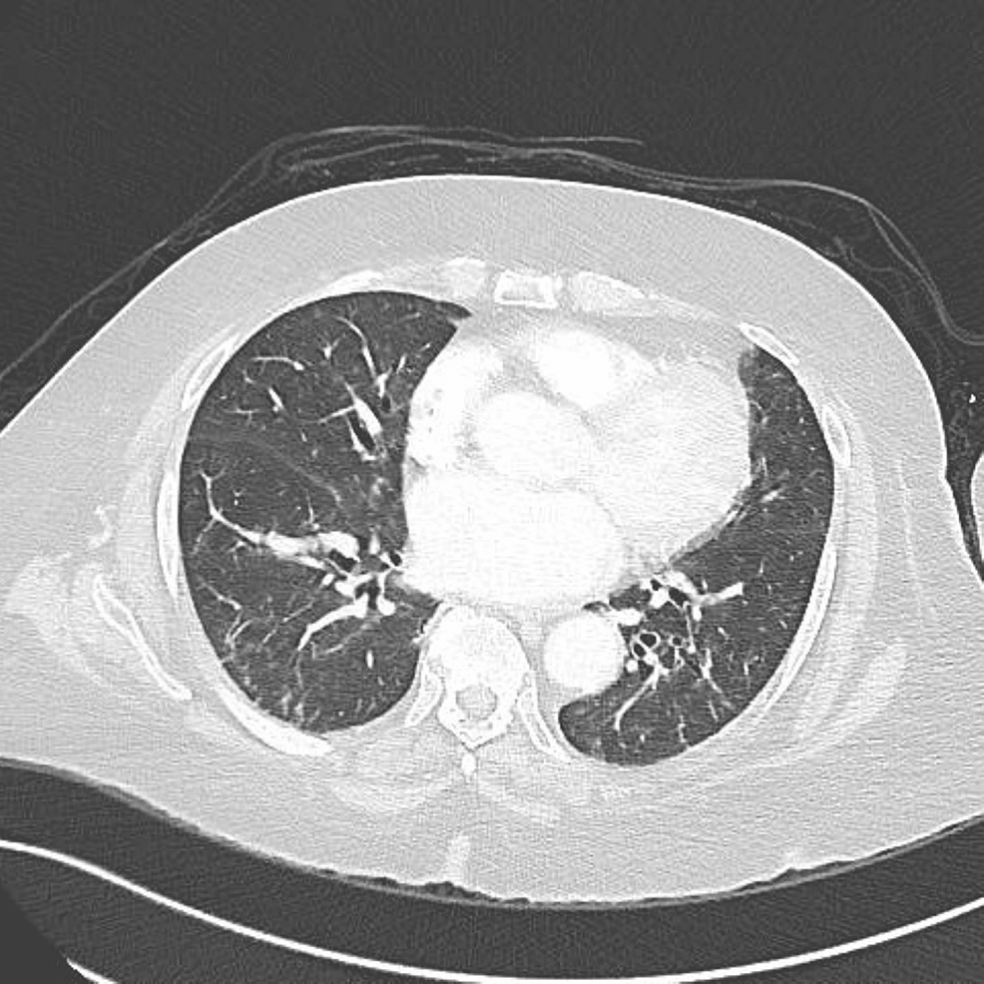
A follow-up CT thorax after four weeks showing almost complete resolution of the reticular and ground glass infiltrates with a residual mild traction bronchiectasis (middle and lower lobes)
